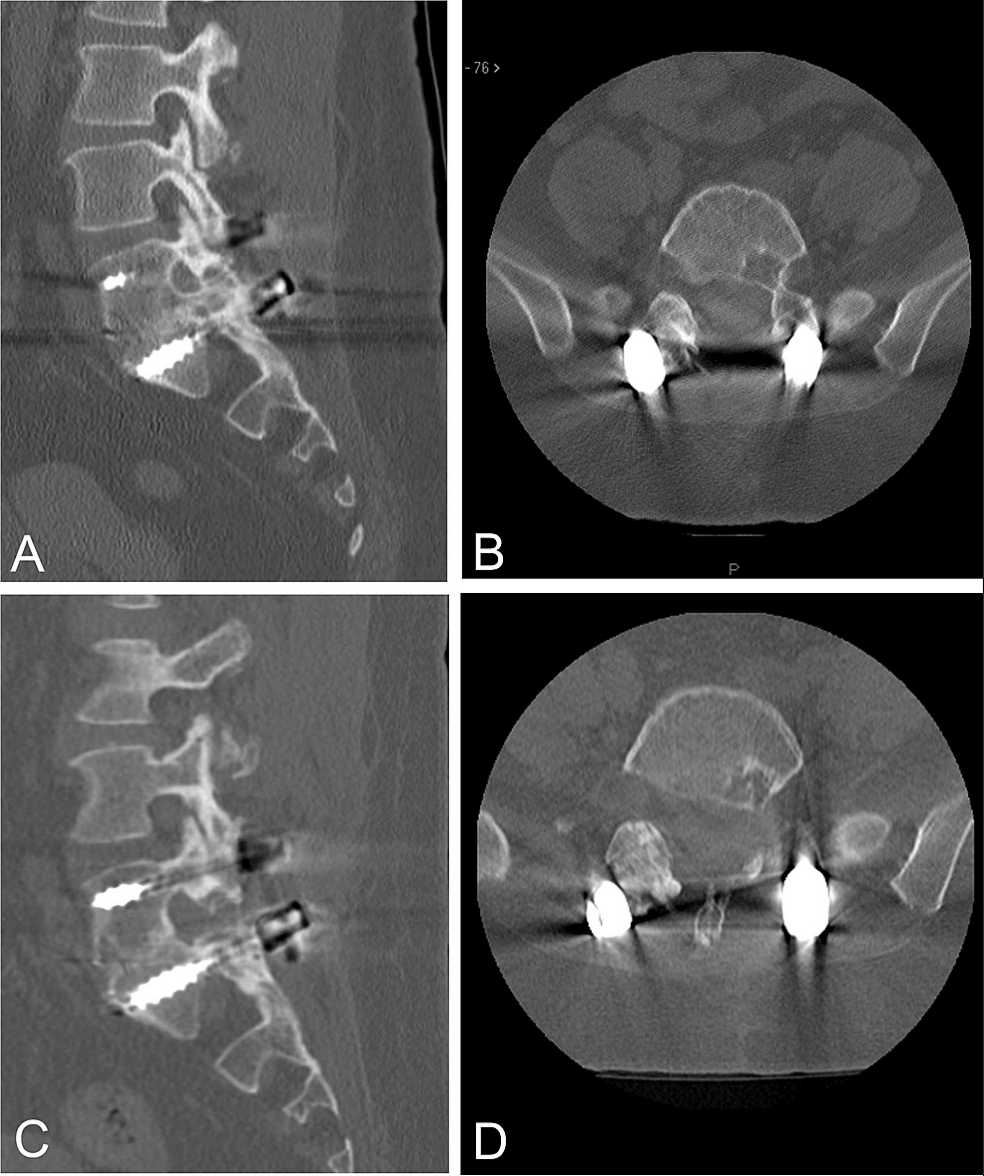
Pre- and postoperative sagittal and axial CT images Preoperative sagittal (a) and axial (b) CT images demonstrating left L5 neuroforaminal stenosis in a patient with recurrent radiculopathy following rhBMP-2 use in spinal fusion. Postoperative sagittal (c) and axial (d) CT images demonstrating appropriate decompression of HO in the left L5 foramen with minimal residual ectopic bone CT: computed tomography; HO: heterotopic ossification; rhBMP-2: recombinant human bone morphogenetic protein-2
